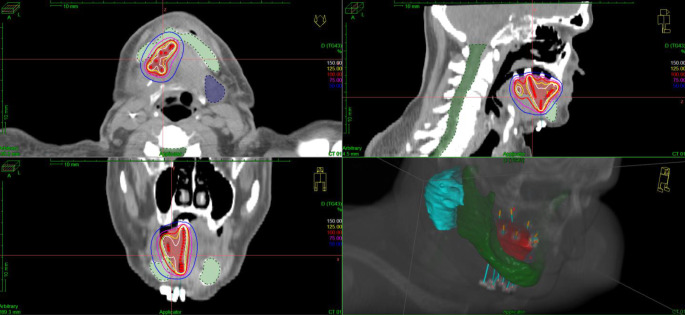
Dose distribution in relation to planning target volume (PTV, *red volume*) in axial, coronal and sagittal views, and three-dimensional computed tomography (3D-CT) reconstruction of the implanted catheters (*blue lines*) and patient anatomy on bottom right
